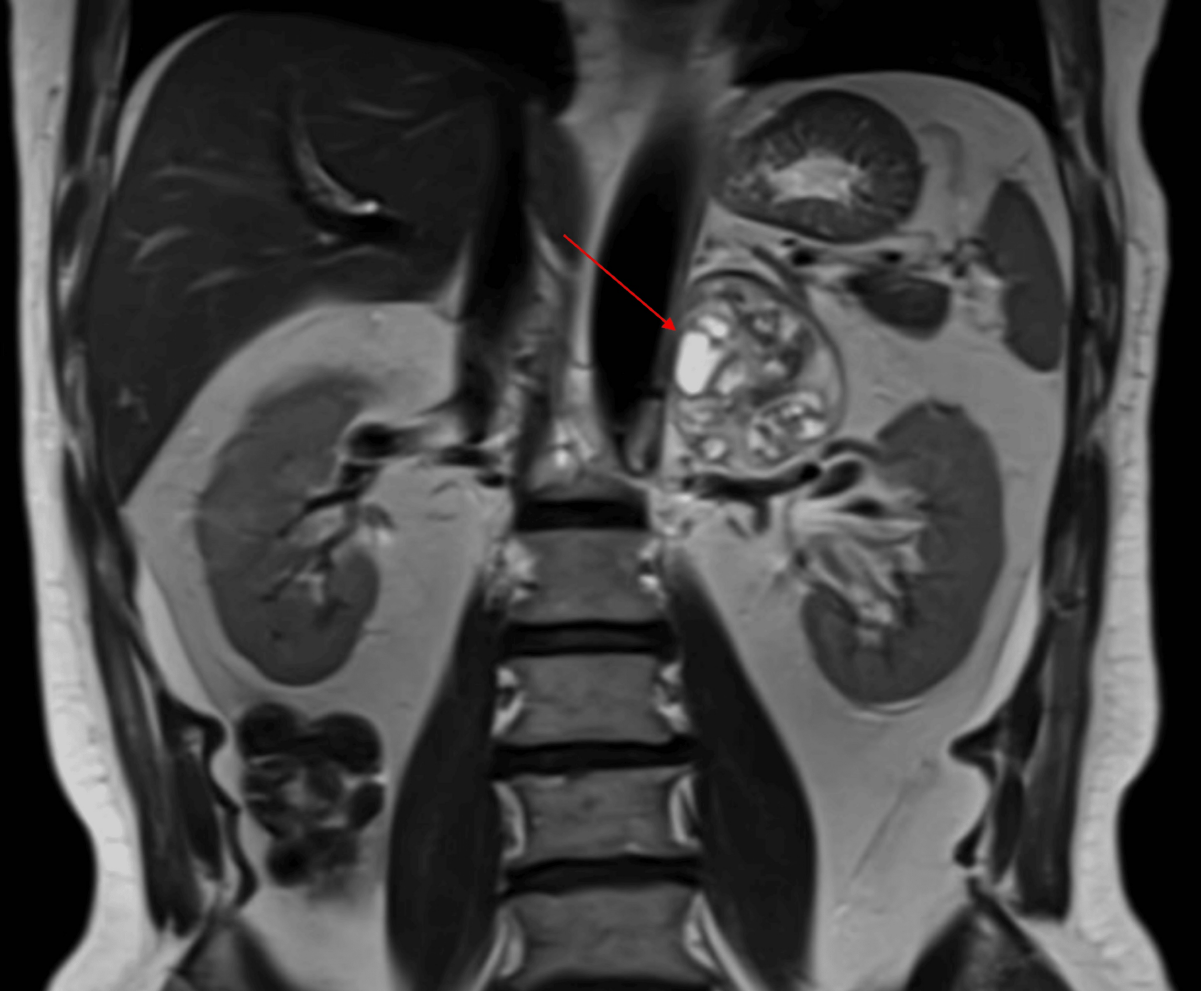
Coronal abdominal MRI showing a left adrenal incidentaloma (arrow) adjacent to the left kidney, later confirmed as an adrenal schwannoma following adrenalectomy.
